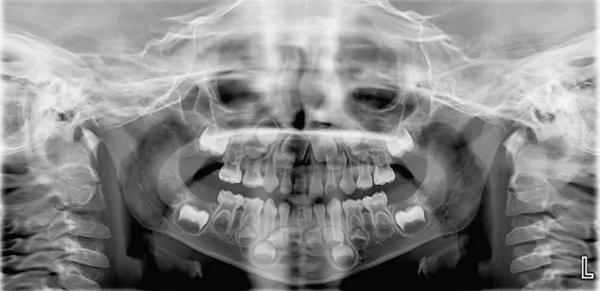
Initial radiograph showing multiple carious lesions in primary teeth.
